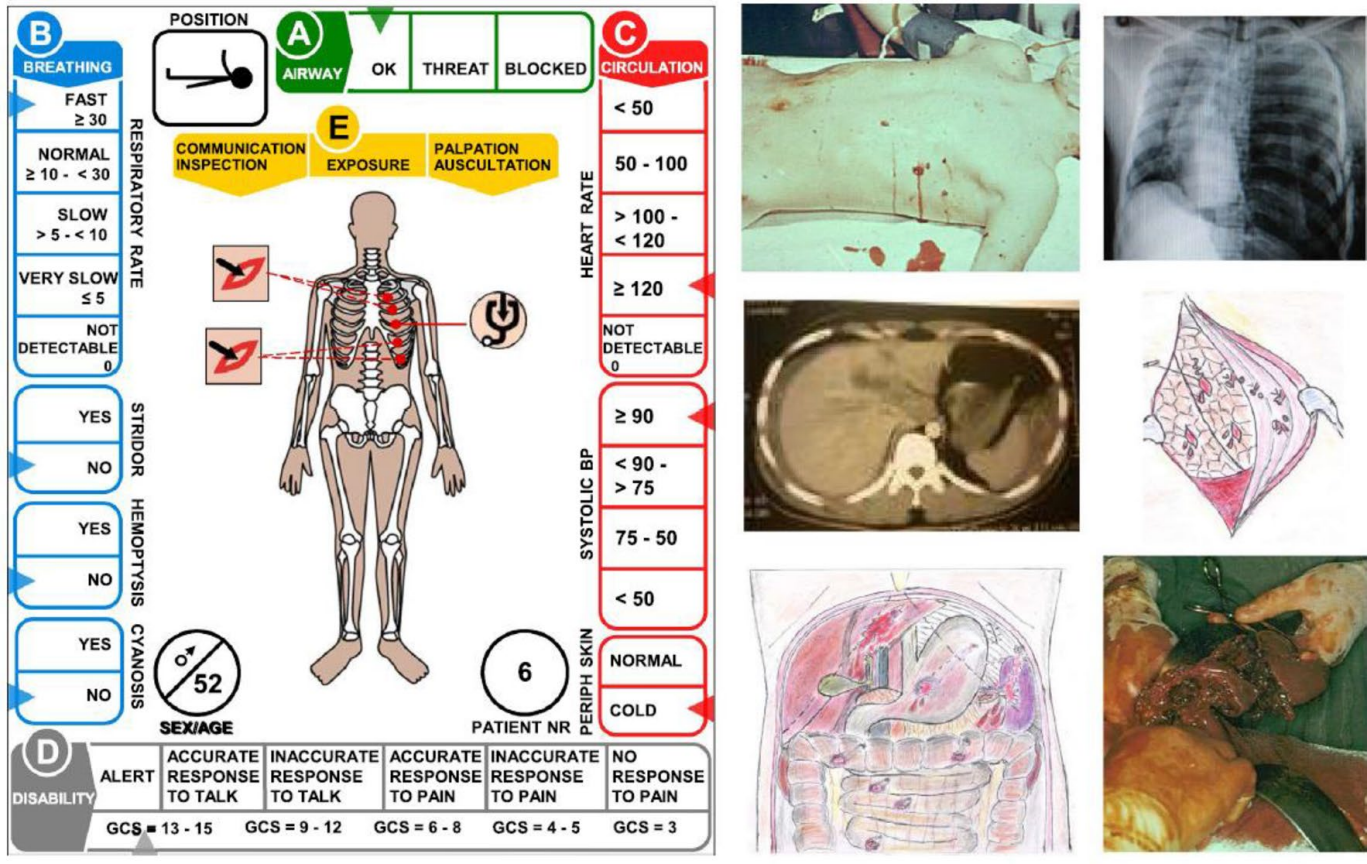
The casualty cards used in this study (for description, see text) were based on real patients from real scenarios. The cards were connected to data files with live pictures, X-ray—and surgical findings as base for decisions with regard to treatment. For each patient, the instructors had access data regarding treatments that had to be done within a certain time to avoid mortality. This made it possible to determine the outcome as a result of the response and of the methodology used
